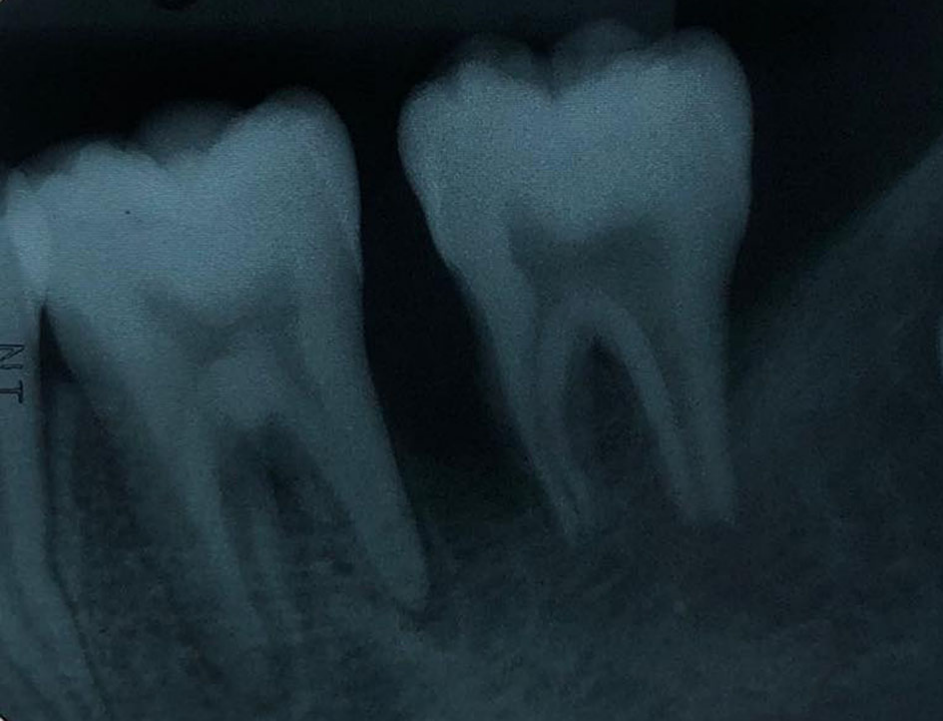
Radiographic examination. The periapical radiograph demonstrating alveolar bone loss at tooth no. 36 and tooth no. 37.
